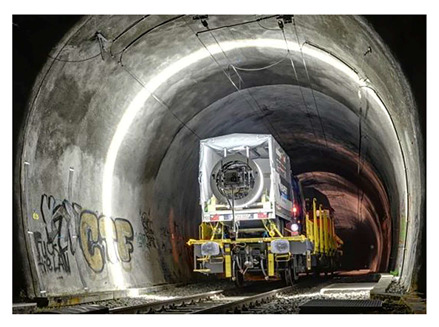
ROBO-SPECT (developed by the ROBO-SPECT Consortium, coordinated by the Institute of Communication and Computer Systems, Athens, Greece) [30]	Multisensor	CCD cameras, laser illumination system, ultrasonic sensor	—	0.5 mm	Spalling
